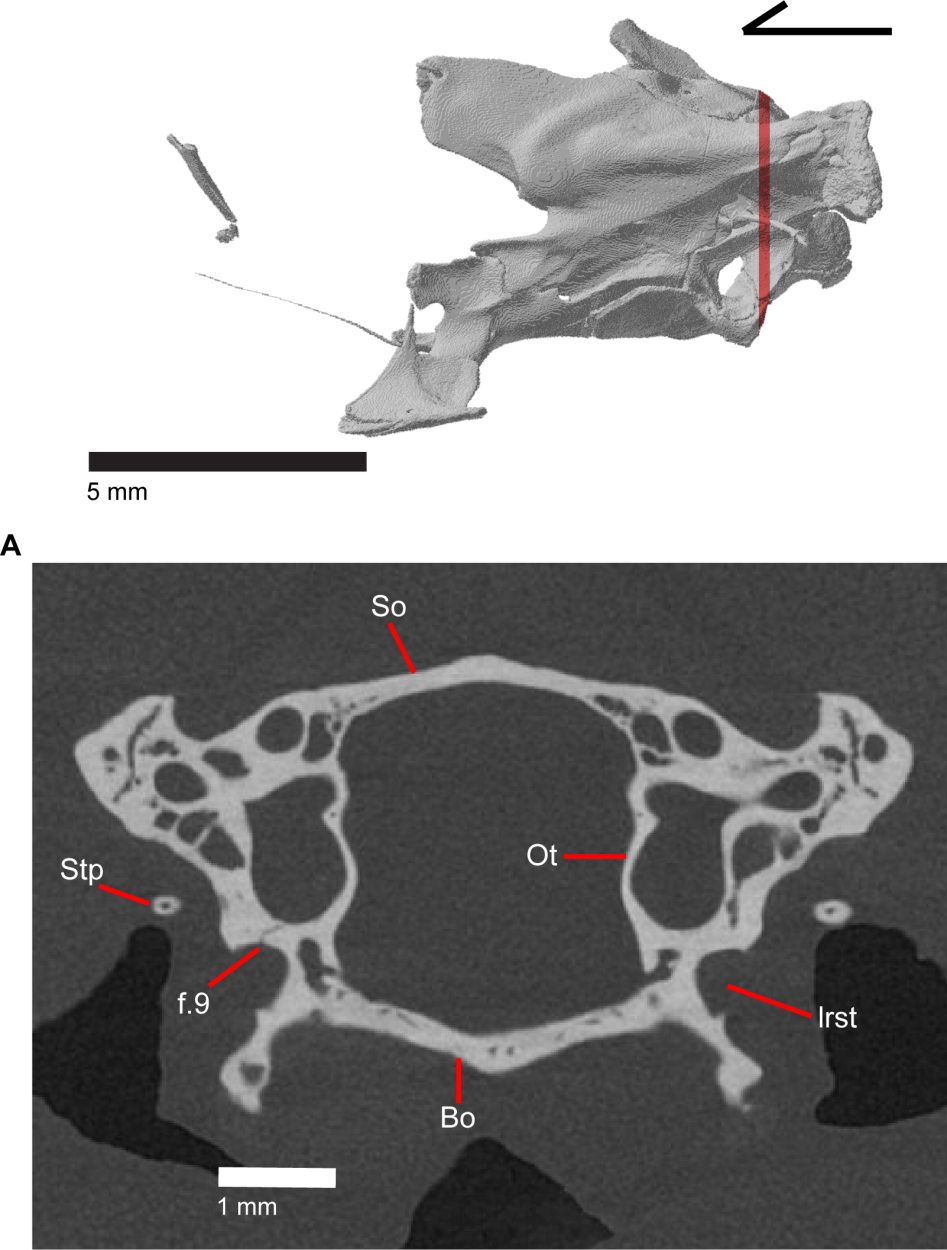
CT coronal slice #1440 of *Elgaria panamintina* MVZ 75918.
